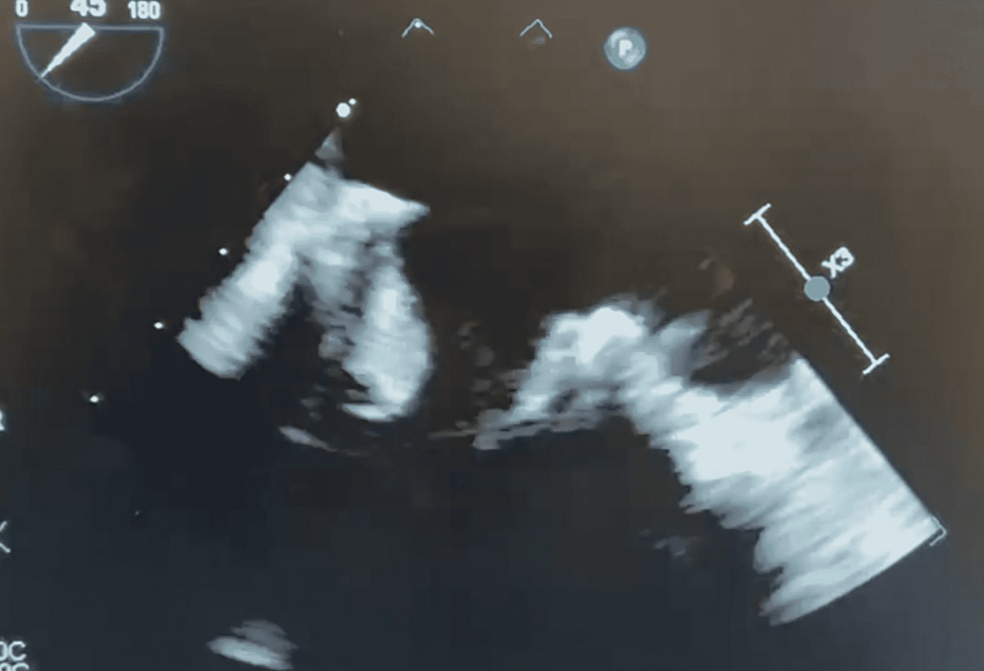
A transesophageal echocardiogram demonstrating a new 1.5 cm vegetation on the bioprosthetic mitral valve
